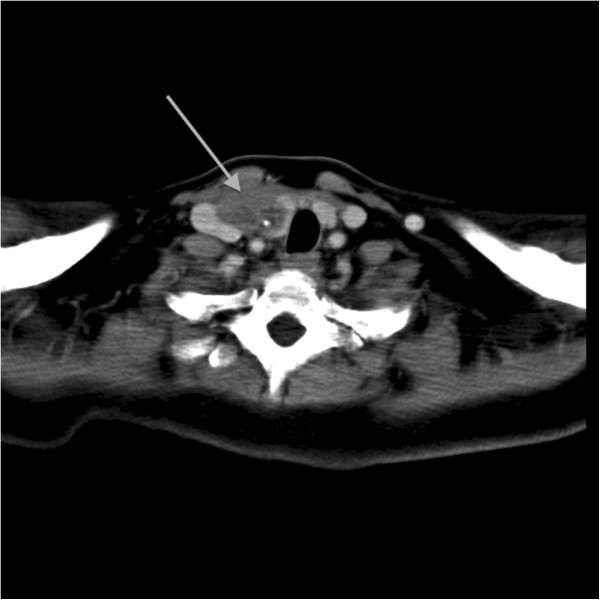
Pre-operative computed tomography of non- functional parathyroid carcinoma (arrowhead) displacing the trachea and the jugular vein, in the right anterior cervical area.
